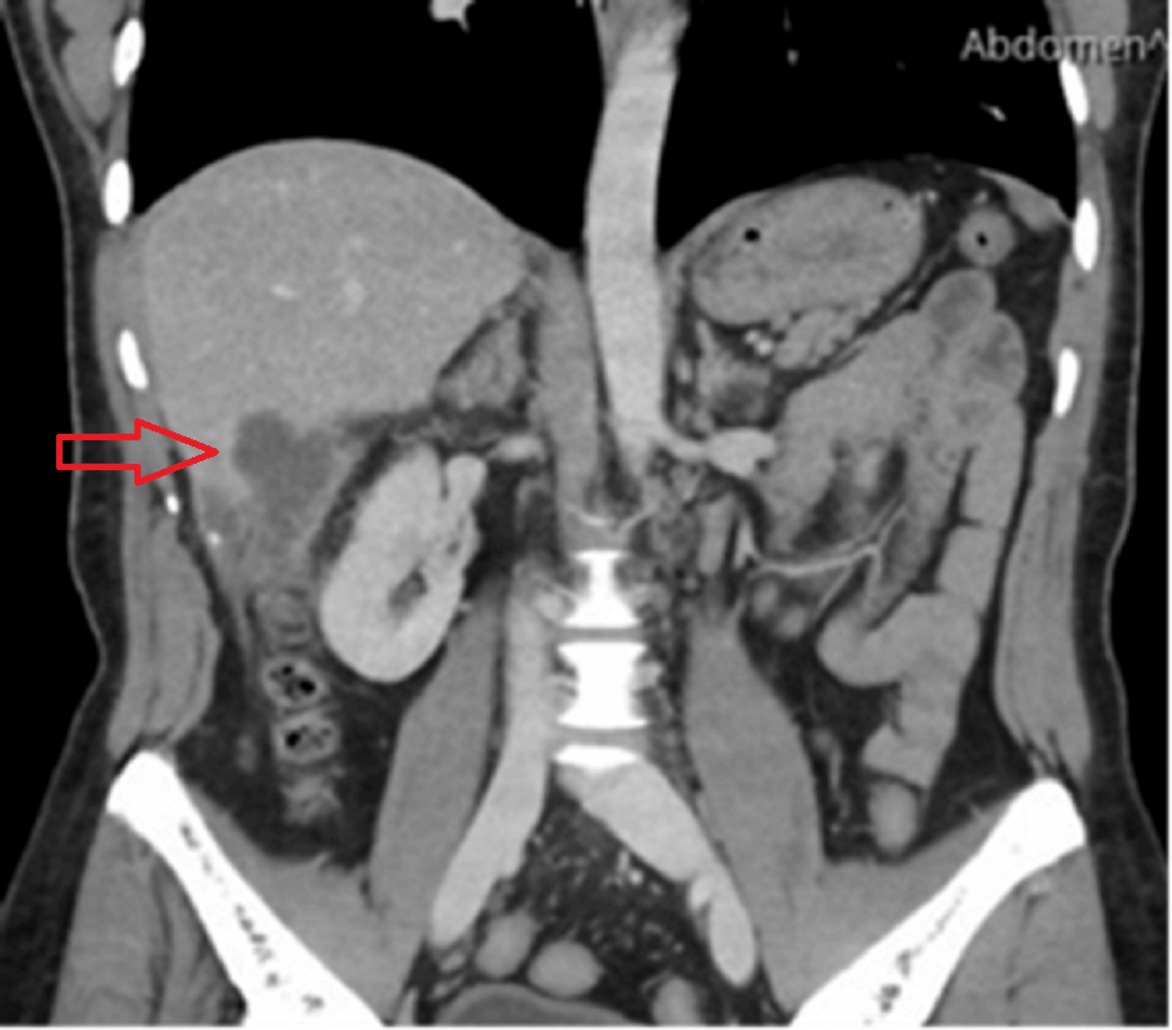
A CT scan of the abdomen demonstrating a subhepatic abscess measuring 2.5 × 4.0 cm with extension to the ascending colon The lower density area, as indicated by the arrow, corresponds to a fluid collection consistent with abscess formation.
